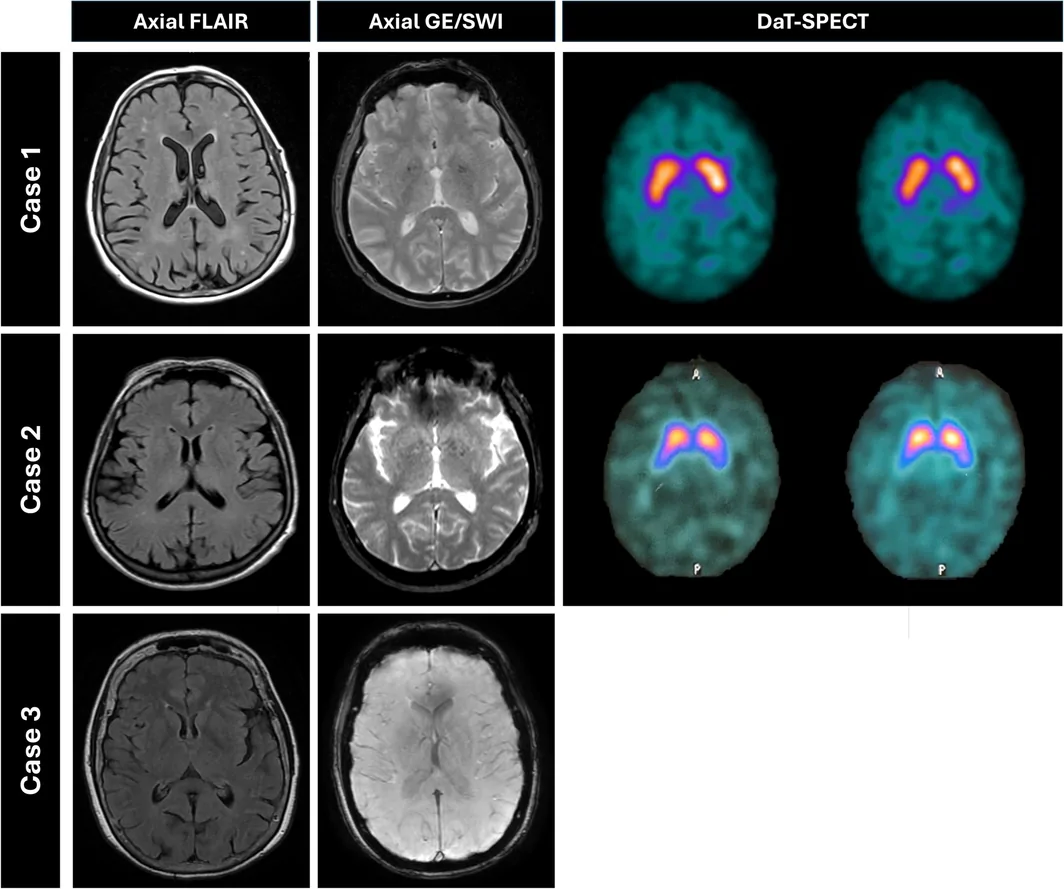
Brain MRI and DaT‐SPECT data in the three cases. Axial fluid‐attenuated inversion recovery (FLAIR) sequences, revealing mild white‐matter hyperintensities without focal abnormalities in all three cases. Gradient‐echo/susceptibility‐weighted imaging (GE/SWI) sequences, revealing minimal basal ganglia iron deposition in cases 1 and 2. DaT‐SPECT images demonstrating a normal presynaptic dopaminergic uptake pattern with no evidence of nigrostriatal denervation.
